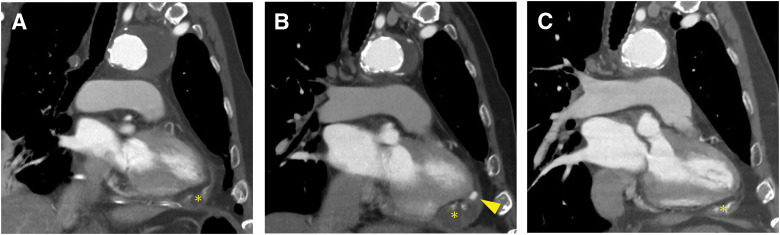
Comparison of perioperative CT. (A) CT on POD 8 shows only minimal pericardial fluid (asterisk) without significant extravasation. The apex repair site was thinned, with a wall thickness of 3 mm. (B) At 3 months postoperatively, a left ventricular pseudoaneurysm (arrowhead) with an associated fluid collection (asterisk) is evident. (C) Follow-up imaging at 3 months after discontinuation of rivaroxaban demonstrates resolution of the pseudoaneurysm and reduction of the fluid collection (asterisk). The thickness of the apical repair section was increased to 6 mm.
